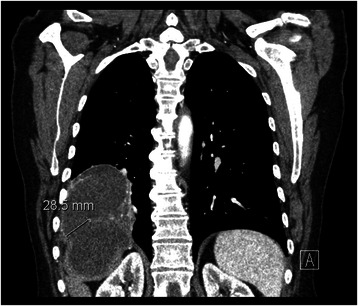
Computed tomography scan demonstrating a complex pulmonary mass excavating anatomical margins and extending into the right hemidiaphragm
